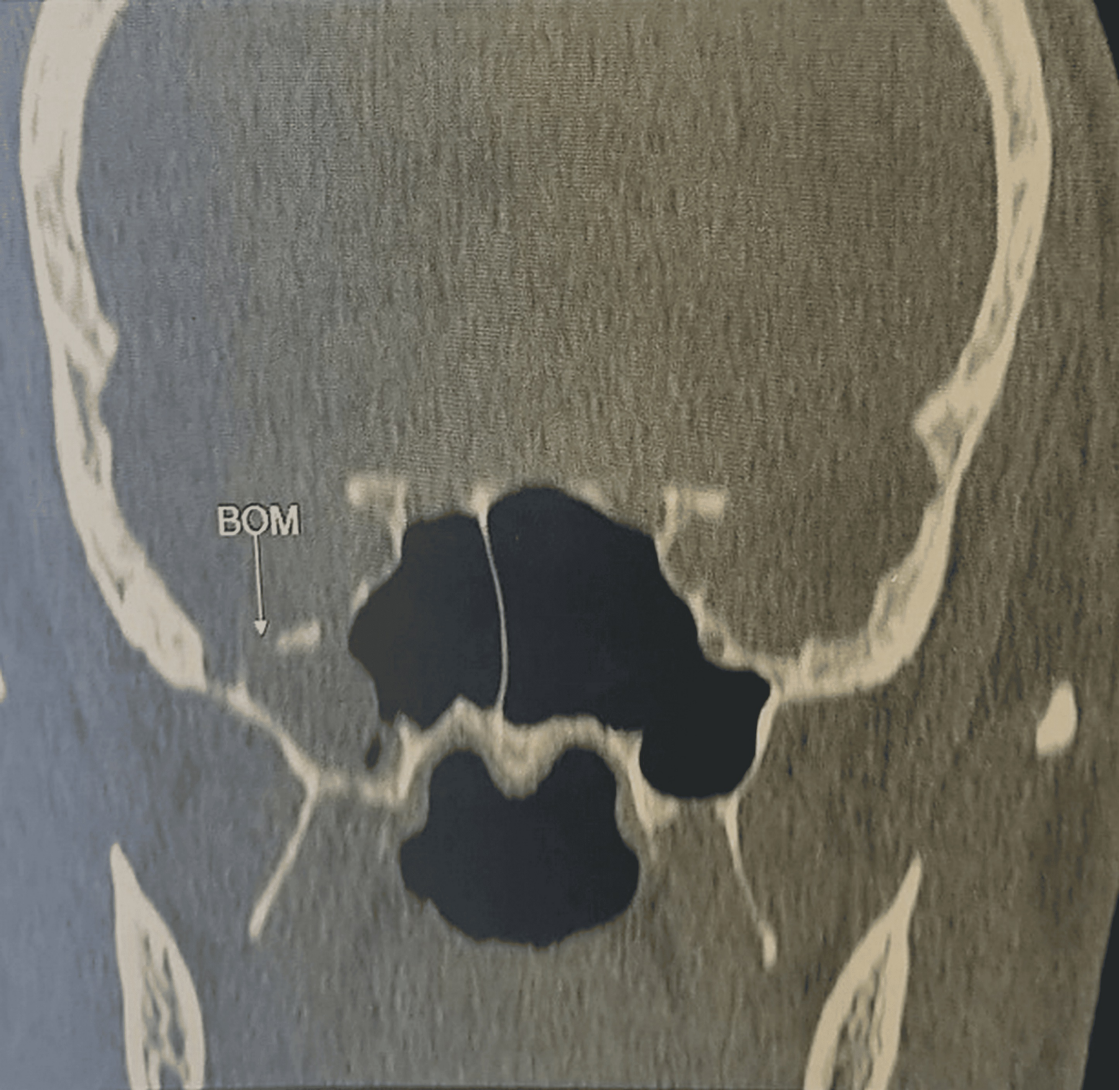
Axial CT scan showing a bony defect in the lateral wall of the sphenoid sinus with herniation of cerebro-meningeal tissue (arrow) CT: computed tomography
